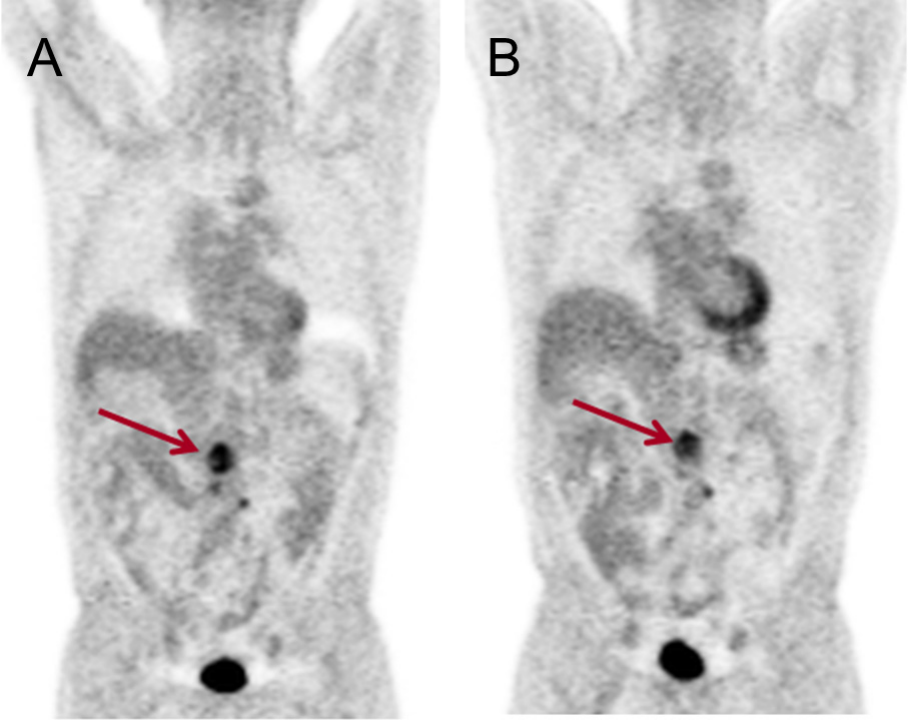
Baseline and post-cycle 2 FDG PET demonstrates improved FDG activity within several abdominal foci at the interim therapy scan (red arrows). Patient experienced PFS at 12 months clinical evaluation. Size measurements on post-cycle 2 MRI (not shown) depicted lesion shrinkage.
